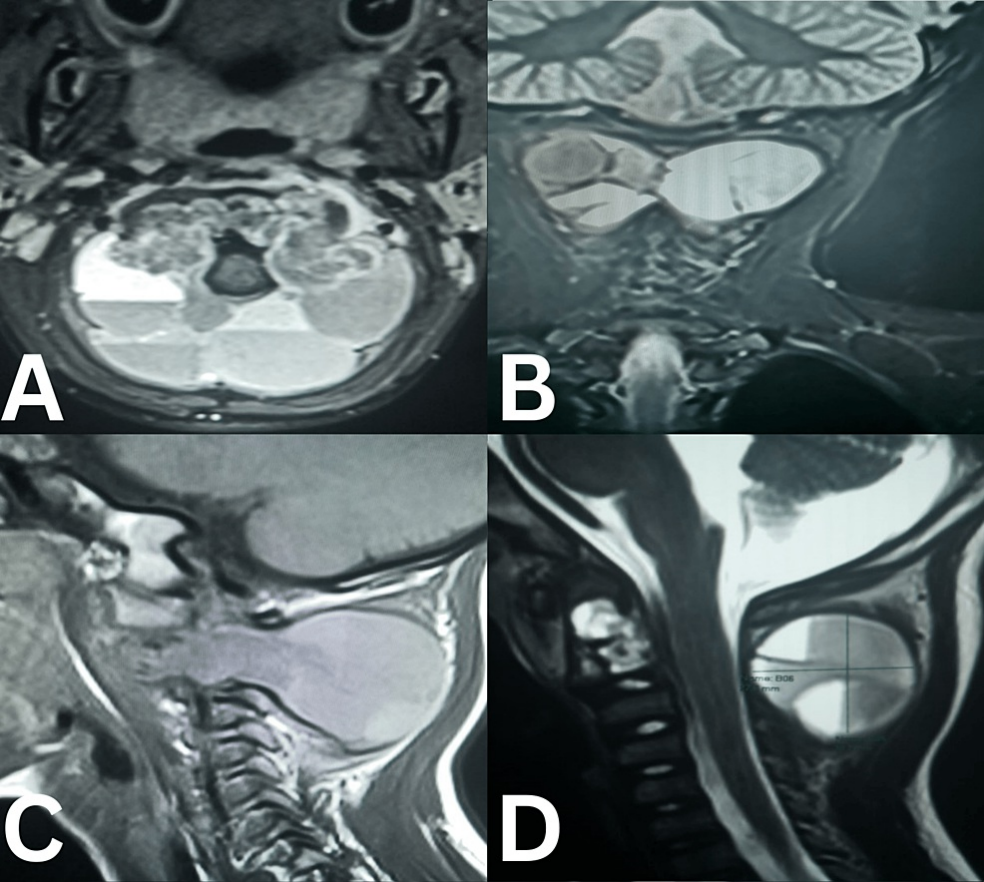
Neck MRI. (A) Axial T2WI demonstrating a large expansile bony lesion with multiple fluid-fluid levels due to blood-filled cavities, separated by septa. (B) T2-W MRI coronal image with variable intensities. (C) T1-W MRI sagittal brain with neck large cyst. (D) T2-W MRI sagittal view with the same finding as in panel C.
